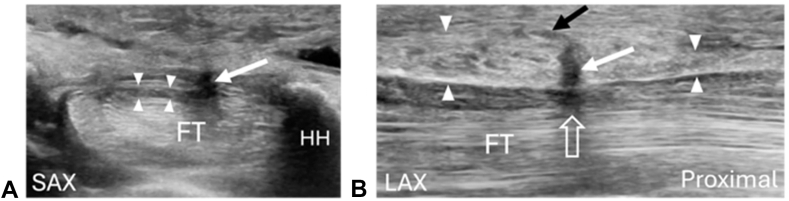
Prerelease images. Images of the carpal tunnel prior to the ultrasound-guided CTR. A Short axis (SAX) or anatomic transverse image of the midcarpal tunnel at the level of the hook of the hamate (HH) showing a hypoechoic and flattened MN (arrowheads) and a hypoechoic appearing TLB (arrow) arising from the ulnar side of the MN and traversing through the TCL complex and into the subcutaneous tissue. The TLB is represented by the vertical shadow, similar to the ultrasound appearance of a TMB. Note that the TLB of the MN is on the ulnar aspect of the nerve which potentially increases the risk of iatrogenic injury during a CTR. FT, flexor tendons. Left = radial, Top = superficial. B Long axis (LAX) or anatomic sagittal image of the MN within the CT showing narrowing (i.e., “hourglass deformity”) of the MN within the midcarpal tunnel (open arrow) and the TLB arising from the MN and passing through the TCL complex (white arrow) and into the subcutaneous tissue (black arrow). The TCL complex is outlined by the arrowheads.
